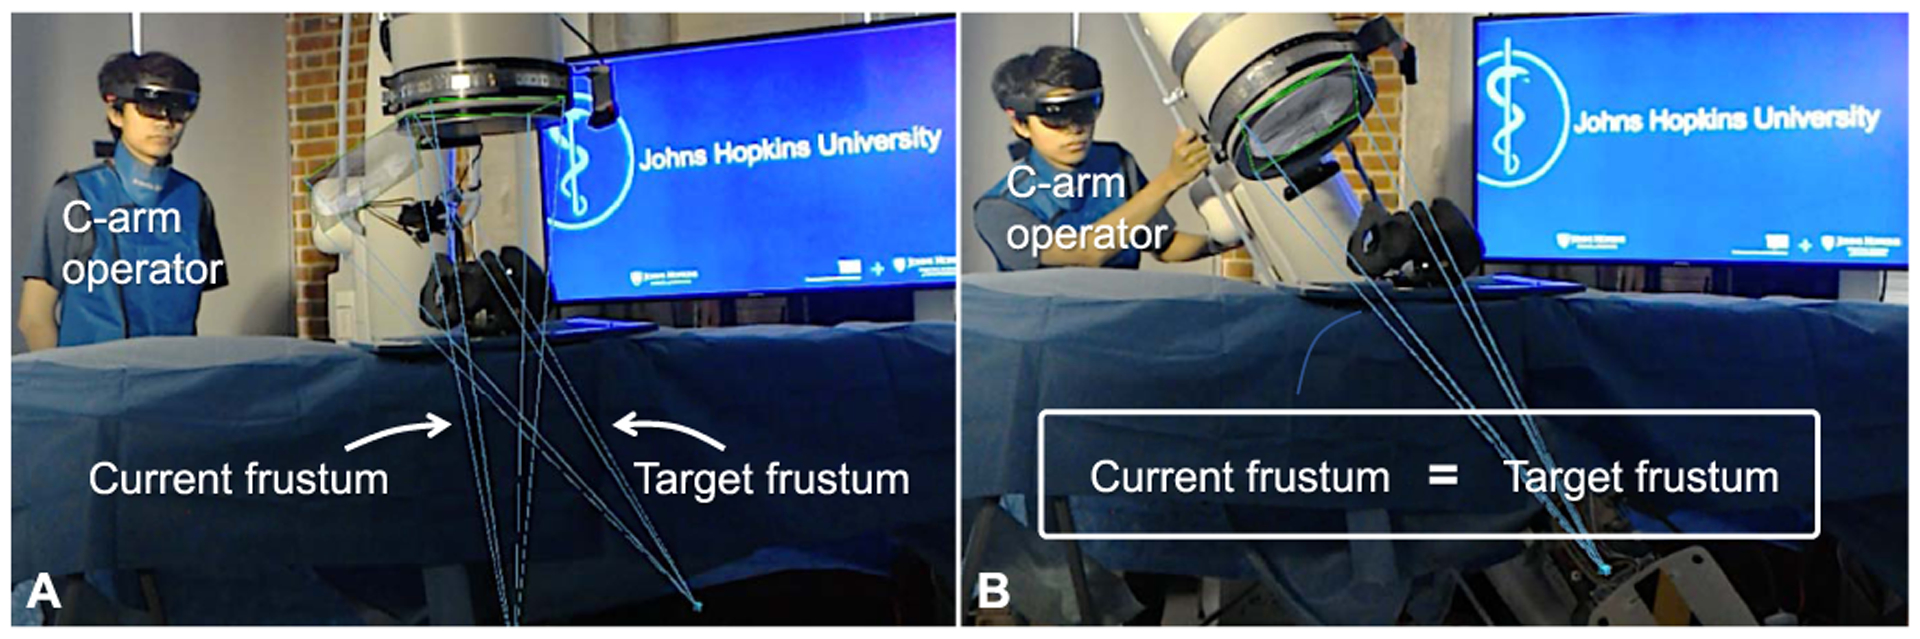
Visualization of a target frustum (A) allows the C-arm operator to align the current C-arm frustum with the surgeon’s desired perspective (B) and eliminate the waste of time and radiation during fluoro hunting. This concept is an example of the capabilities of interactive frustums on moving information between different stake holders in the OR, *i.e.* surgeon, patient, X-ray technician, staff, etc.
